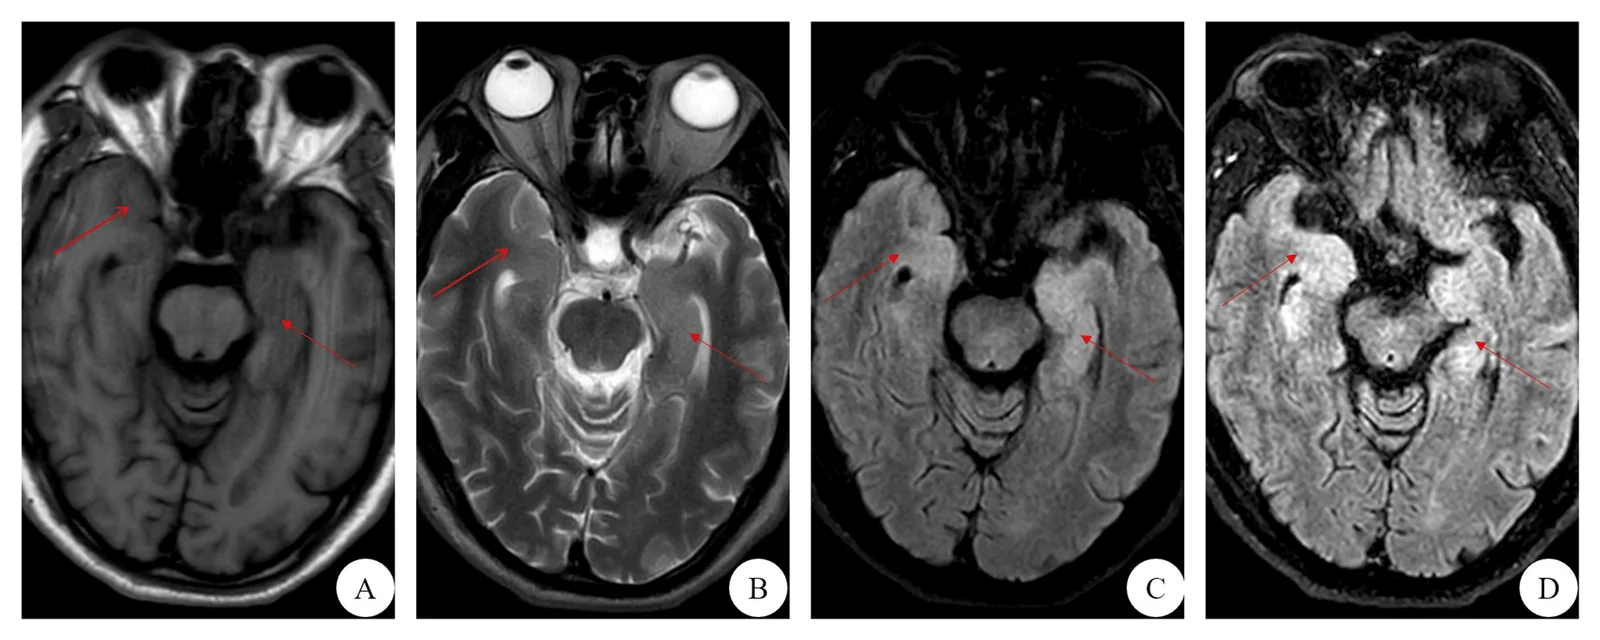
Brain MRI findings (A) In the T1-weighted image, the bilateral hippocampal lesions show slightly hypointense signals (the red arrow). (B) In the T2-weighted image, the lesions appear as hyperintense signals (the red arrow). (C) In the FLAIR image, the lesions also present as hyperintense signals (the red arrow). (D) In the enhanced scan image, there is no significant enhancement of the lesion (the red arrow). Bilateral hippocampal T2/FLAIR hyperintensity indicates limbic inflammation, which is a characteristic but not universal finding in anti-LGI-1 encephalitis. The absence of contrast enhancement suggests a non-neoplastic, inflammatory etiology rather than a tumor MRI: magnetic resonance imaging; FLAIR: fluid-attenuated inversion recovery; LGI-1: leucine-rich glioma-inactivated 1
